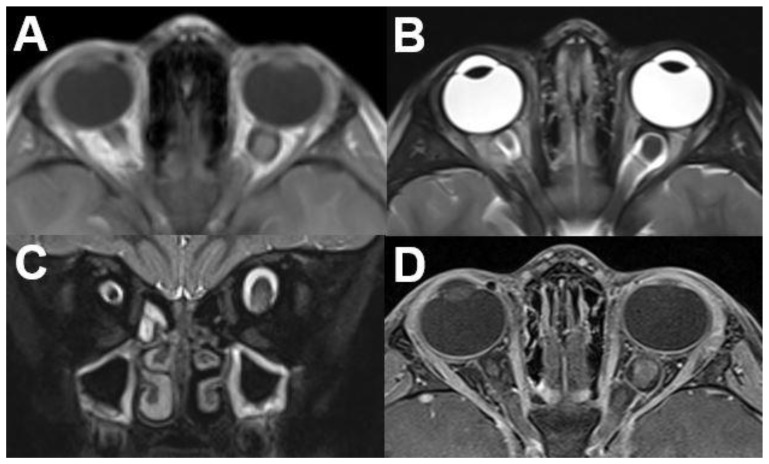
Neurofibromatosis type I (NF1).* MRI of a 5-year-old female with NF1. The left optic nerve is thickened on axial T1-weighted (A) and T2-weighted images in axial (B) and coronal view (C). Additionally, a slight contrast enhancement can be detected (D).
